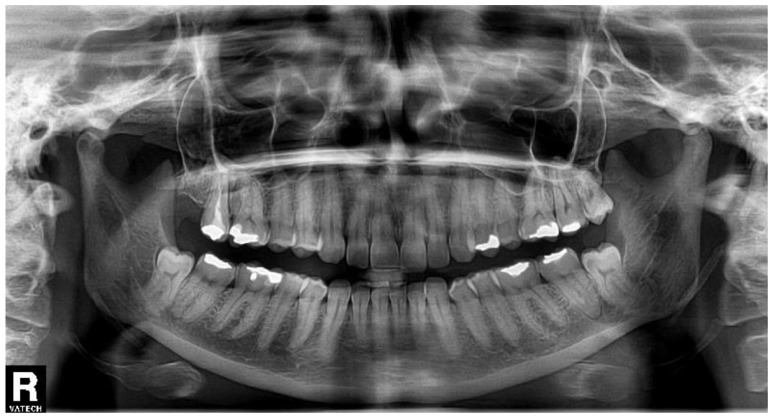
The orthopantomogram of the patient 4. Tooth No. 38 is in infra-occlusion, with a semilunar burnout distally.
